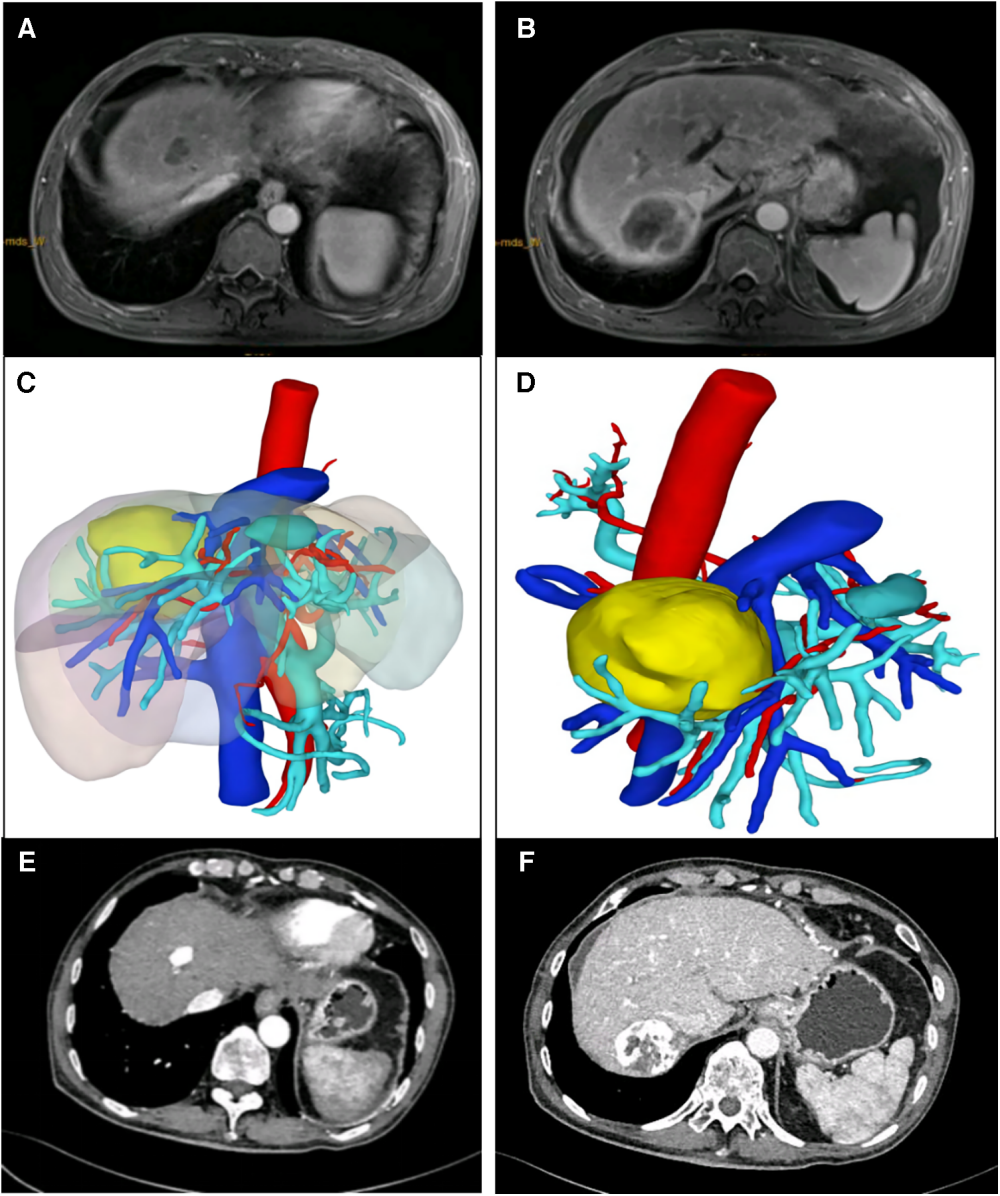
Typical case who received systemic treatment combined with TACE treatment before LH and RFA. (A,B) The main HCC was mainly in segment VII, and a 3 cm lesion was in segment VIII. (C,D) Preoperative 3-D reconstruction model of the patient, and the main HCC was close to the confluence of the right hepatic vein and the inferior vena cava. (E,F) CT image of the patient after 24 weeks of lenvatinib + camrelizumab combined with TACE treatment.
